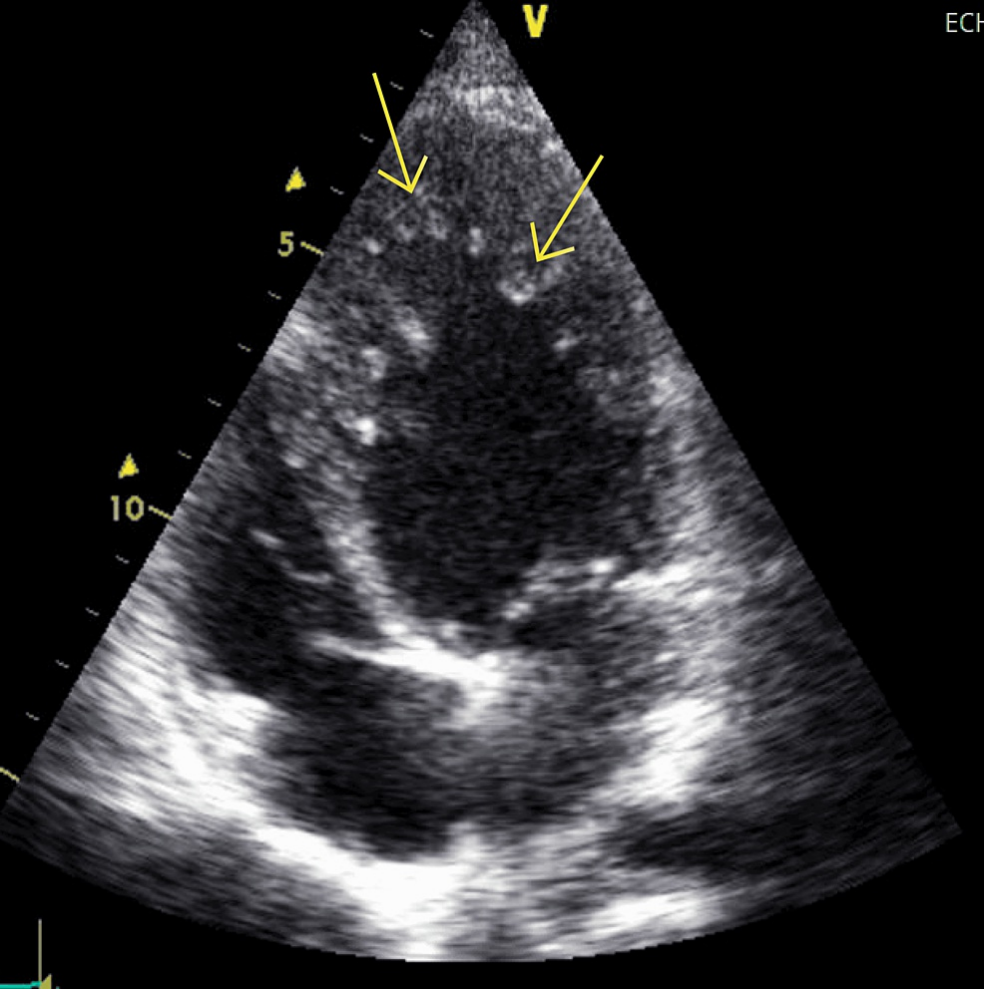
Trans-thoracic echocardiography Normal LV size with prominent trabeculations (arrows) in the LV cavity is consistent with noncompaction cardiomyopathy. LV: left ventricle
